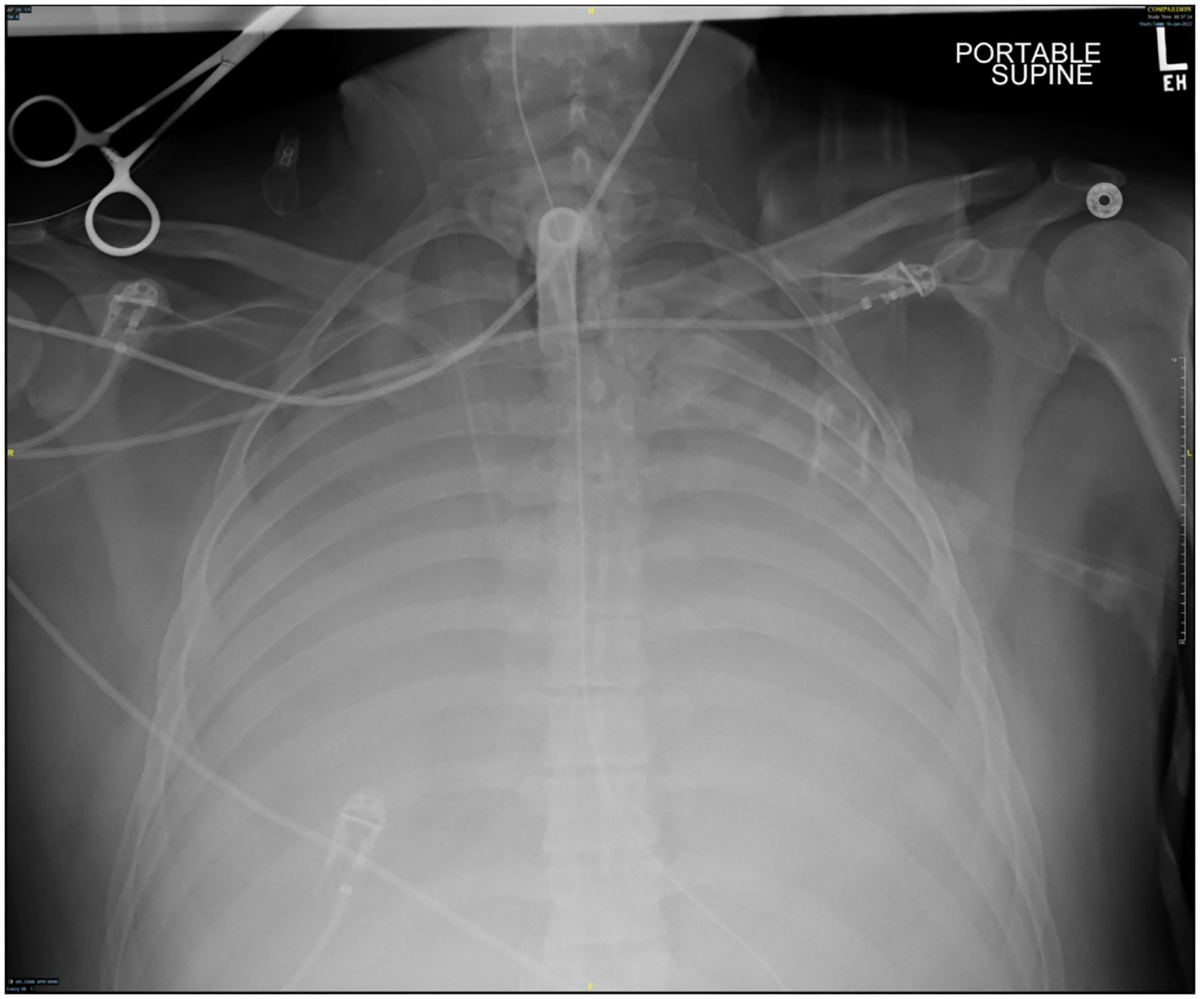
Chest x-ray at the time of hemoptysis.
